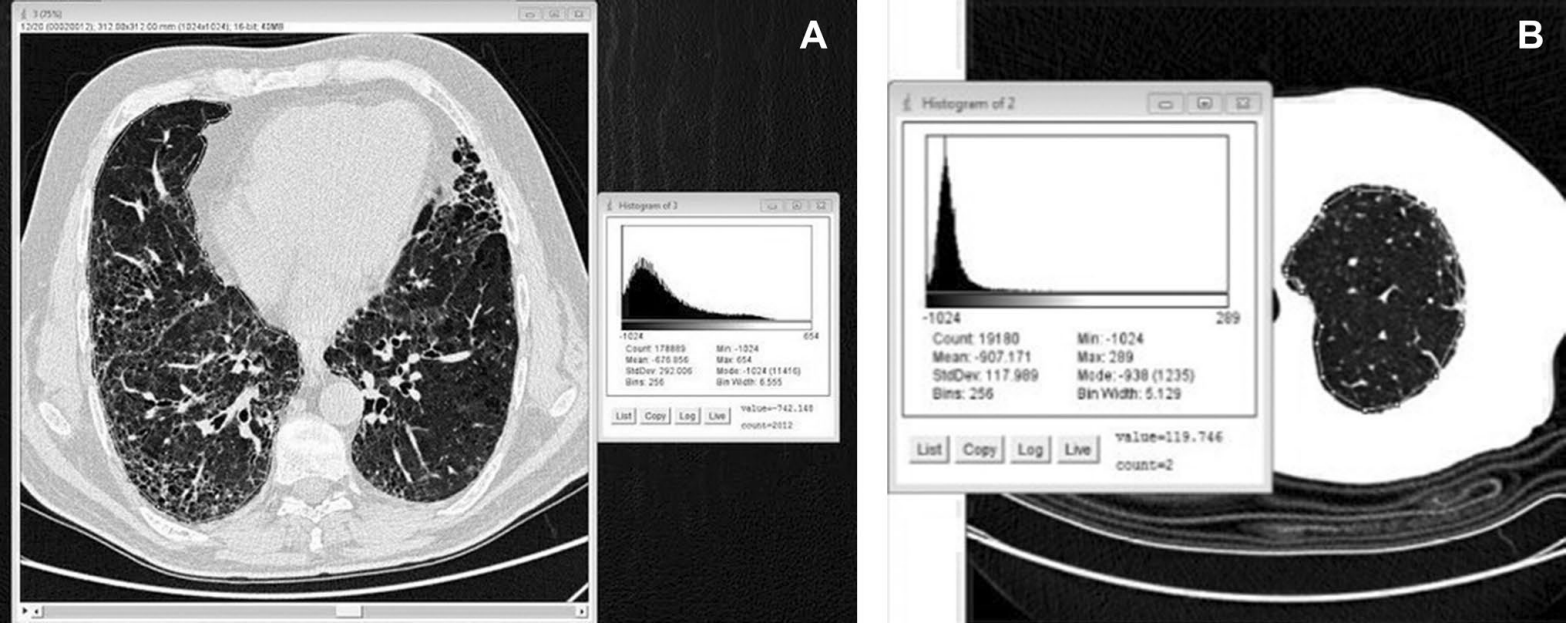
A representative slice segmentation (marked in yellow) of the right lung in a patient affected by advanced idiopathic pulmonary fibrosis and evaluated by means of thin section volumetric CT is shown in panel A. On the right side, results of digital processing analysis of the given slice are reported. After the whole lung sampling was completed, the software automatically generated averaged data from the analysis of all slices of both lungs. The density histogram of the fibrotic lung is both less peaked and less skewed than that of a control normal lung (Gaussian curve sharply shifted to the left with a very narrow and high peak), as reported in panel B for comparison
